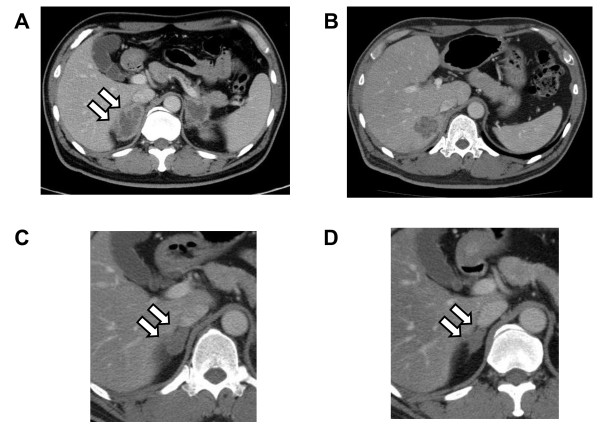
Computed tomography of the abdomen. (A and B) The enlarged bilateral adrenal glands (A) and liver mass (B) before the unilateral adrenalectomy. (C and D) Decreased size of the right adrenal mass at 3 (C) and 12 (D) months after the left adrenalectomy. The arrows show the right adrenal mass.
